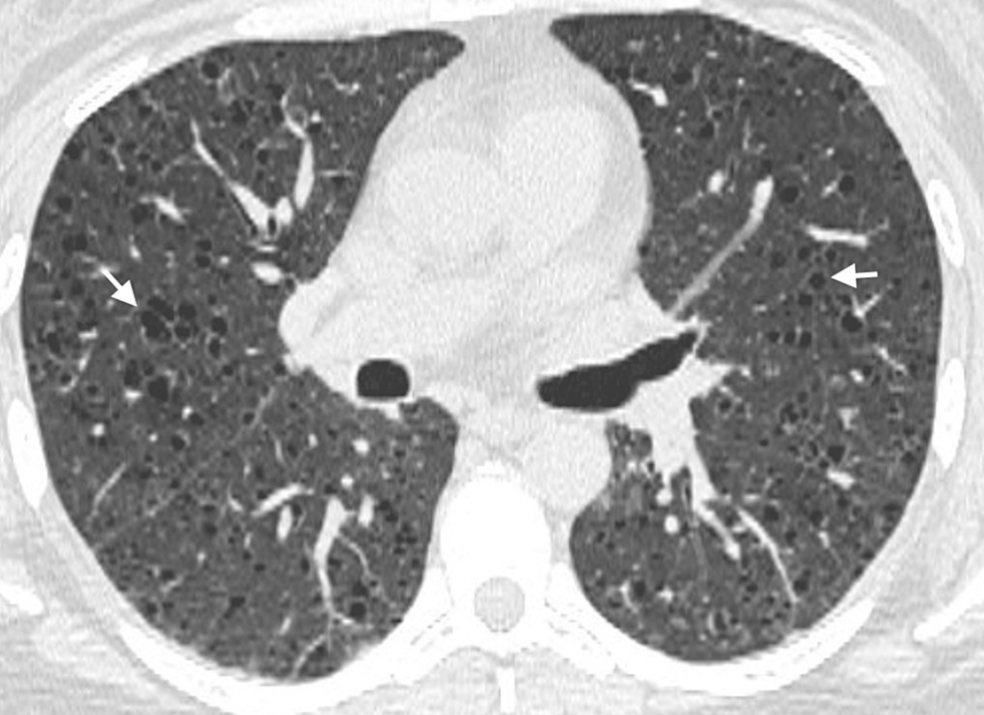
A high-resolution computed tomography of the chest of the patient displayed in the lung window showing small round bilateral cysts (white arrow).
